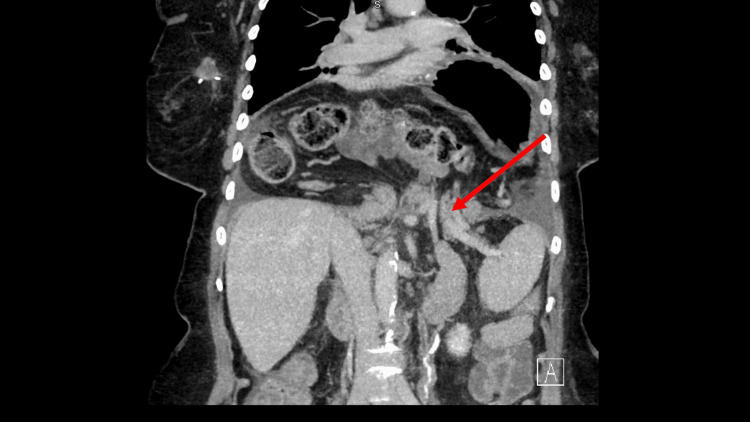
CT taken on readmission day 1 - image 2 The image demonstrated a recurrence of the hiatal hernia containing the majority of the stomach, large portions of small bowel, and transverse colon. Edema was imaged around the pancreatic tail, which was reflective of mild pancreatitis CT: computed tomography
